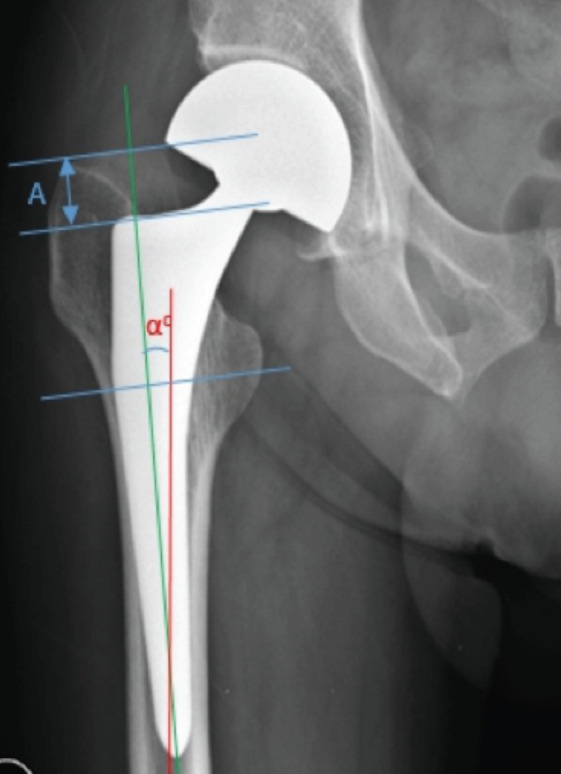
Anteroposterior post-operative radiograph of a right hip showing determination of femoral stem subsidence and alignment of the femoral stem.
